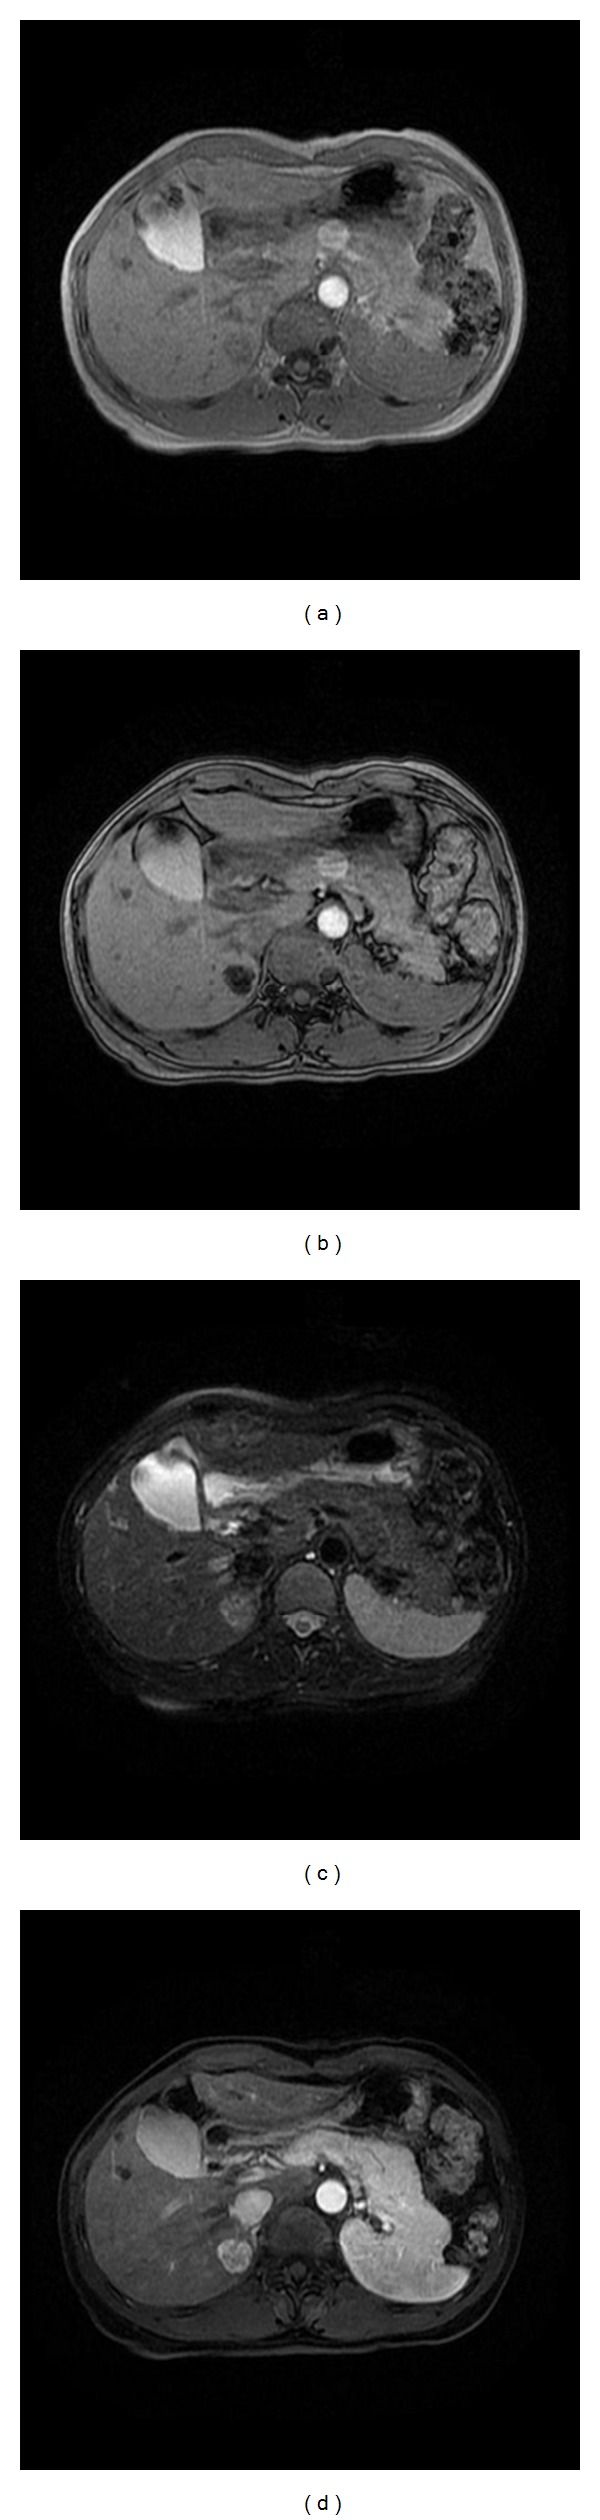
MR findings of the liver mass. On MR T1-weighted in-phase images, the mass shows low signal intensity (a), and on out-of-phase images, the mass shows signal drop and dark signal intensity (b). On MR T2-weighted images, the mass shows high signal intensity (c). The mass demonstrates high signal intensity on arterial phase after contrast injection (d).
